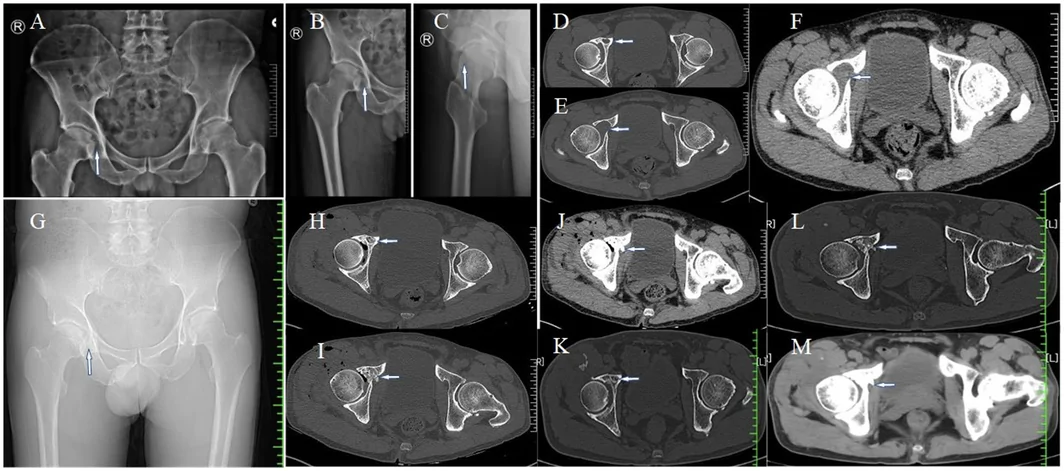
Conventional radiography and computed tomography (CT) of the right hip. (A–F) Preoperative images, (G–J) Immediate postoperative images, and (K–M) Images obtained at the 2‐month follow‐up. Preoperative CT demonstrates erosion and a partial osseous defect of the anterior and inferomedial acetabular wall. Immediate postoperative images demonstrate filling of the acetabular defect with an autologous iliac bone graft. Follow‐up images show maintained graft position and osseous incorporation. The preoperative plain radiograph shows no definite abnormality. Arrows indicate the acetabular lesion or reconstructed defect.
